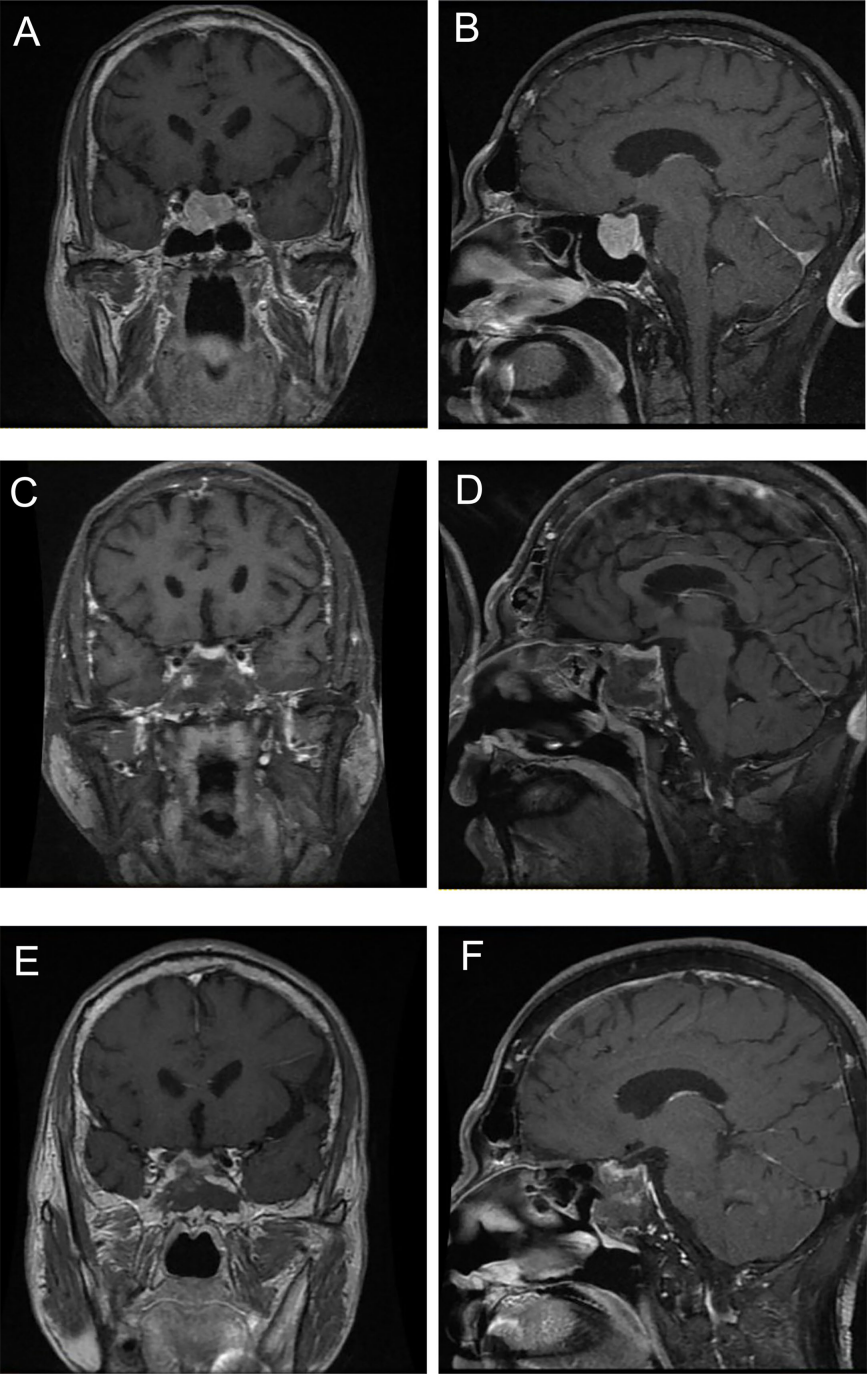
The MRI images of a GH/TSH cosecreting pituitary adenoma at different treatment stages. (A) and (B) were taken before surgery, (C) and (D) were taken at third month after surgery, (E) and (F) were taken at fifth year after radiotherapy.
